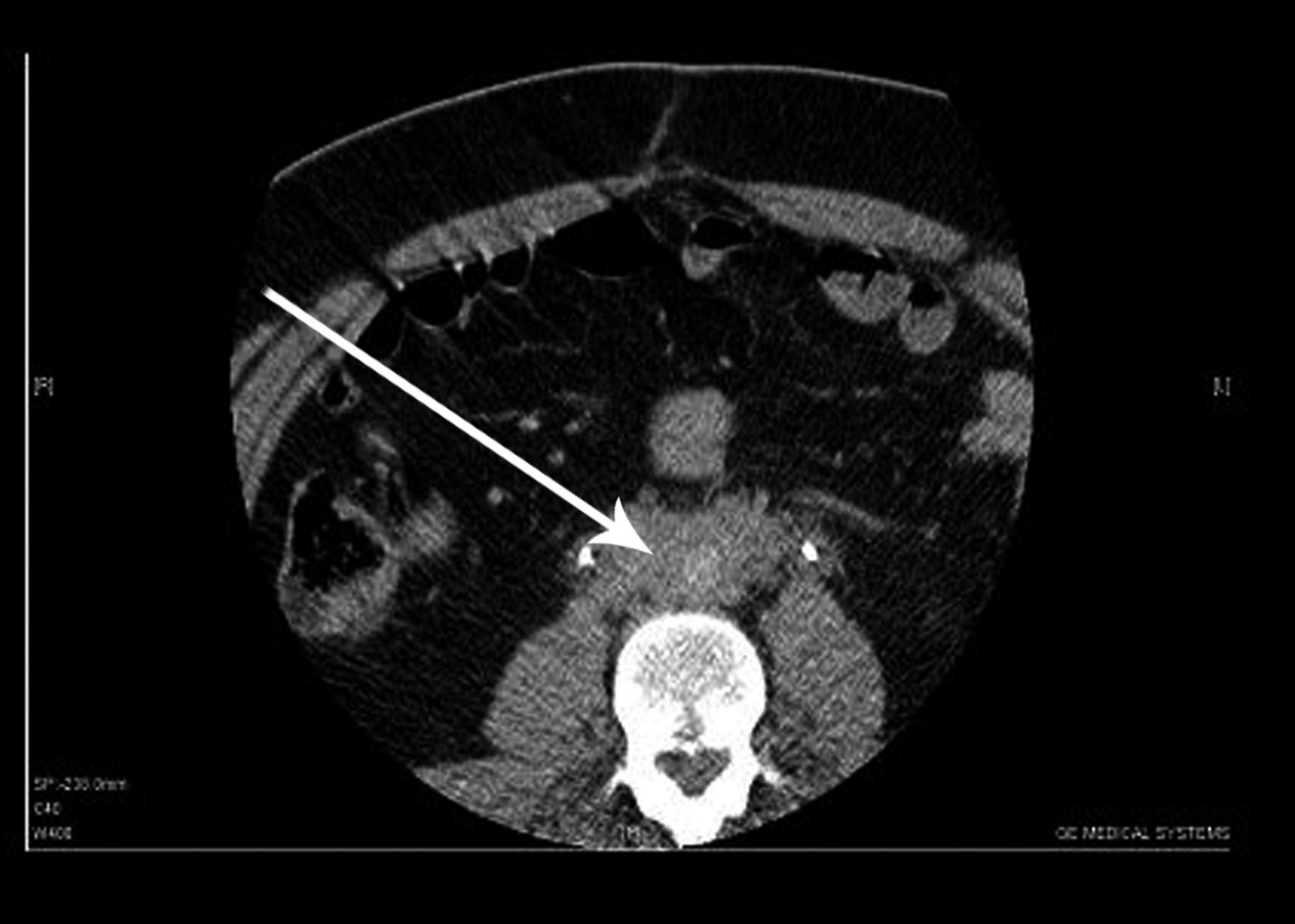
A 40-year-old man presenting with pain in the flank and abdomen together with flank tenderness CT scan without contrast shows a soft tissue mass (arrow) which is located in the retroperitoneal area at the level of the fourth and fifth lumbar vertebrae.
